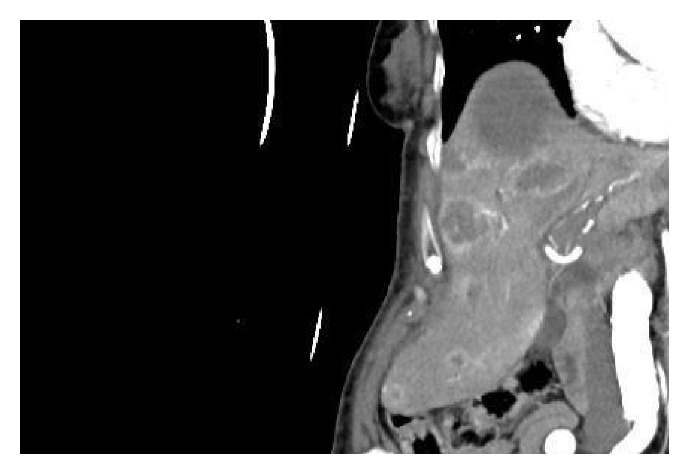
CT scan after endoscopic hemostasis demonstrates liver metastasis and the tumor recurrence in the remnant stomach (frontal image in arterial phase).
